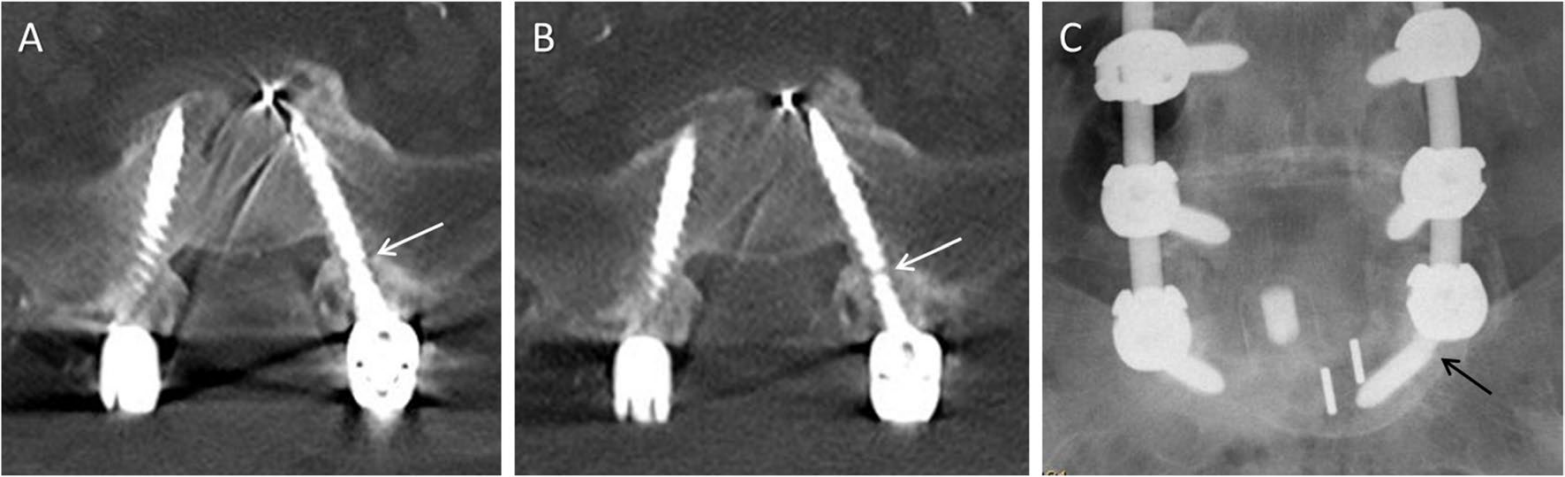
A 78-year-old female with lumbar instrumentation L3-S1. On the reformatted axial CT image of the tin-filtered low-dose CT (B) a fracture of the left S1 pedicle screw (arrow) is visible, which was missed by both readers on the standard CT (A) because of masking due to stronger artifacts. The fracture of the left S1 pedicle screw (arrow) is also visible on the anteroposterior radiograph of the lumbar spine (C)
